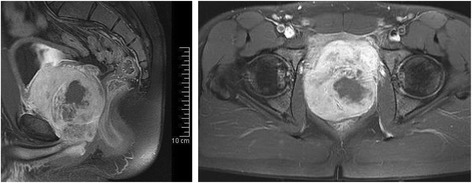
Axial (*left*) and transversal (*right*) section of magnetic resonance imaging (MRI) showing an inhomogeneous tumor mass in the prostate infiltrating the bladder wall and the seminal glands
